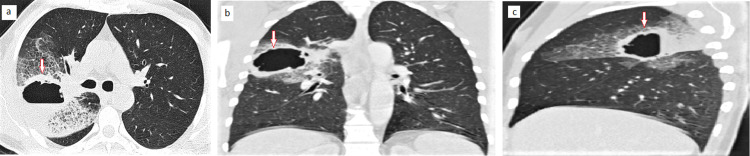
Chest computed tomographic scan axial (a), coronal (b), and sagittal (c) reformatted images, showing a large cavitating mass (arrow) associated with peritumoral reticular infiltrates.
